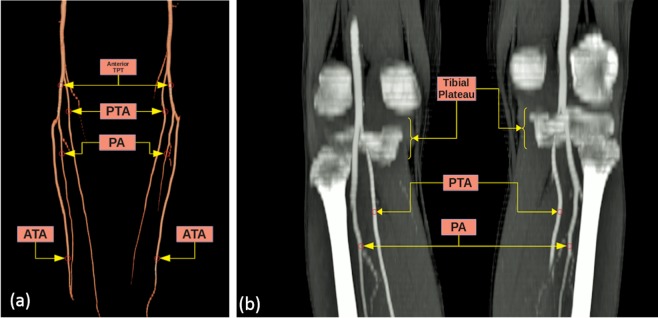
Three-dimensional volume rendered image (a) and the CT angiography image (b) show the Type IIB pattern on both limbs of the same patient. TPT: Tibioperoneal trunk, PTA: Posterior tibial artery, PA: Peroneal artery, ATA: Anterior tibial artery.
